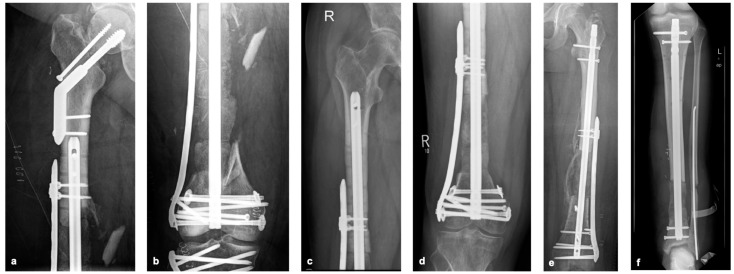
Postoperative X-ray after reconstruction of bone defects of the femur and tibia using the pearl-string technique (PST), case from Figure 4a,b 48 hours after surgery (a,b), other cases (c–f) describing the PST in other patients at 3-month follow-up.
